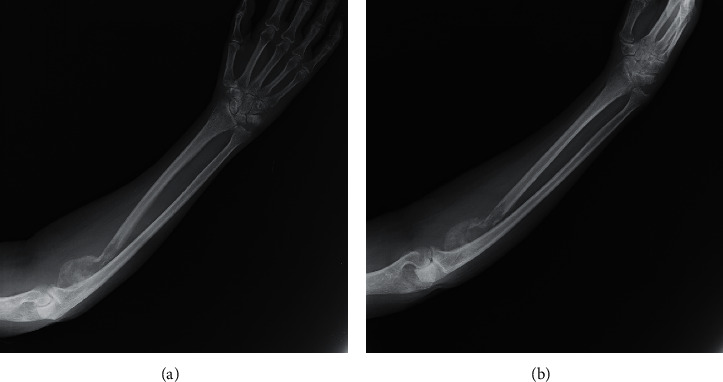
Two radiographs showing bone healing of the pathologic fracture of the right radius at two months of follow-up.
